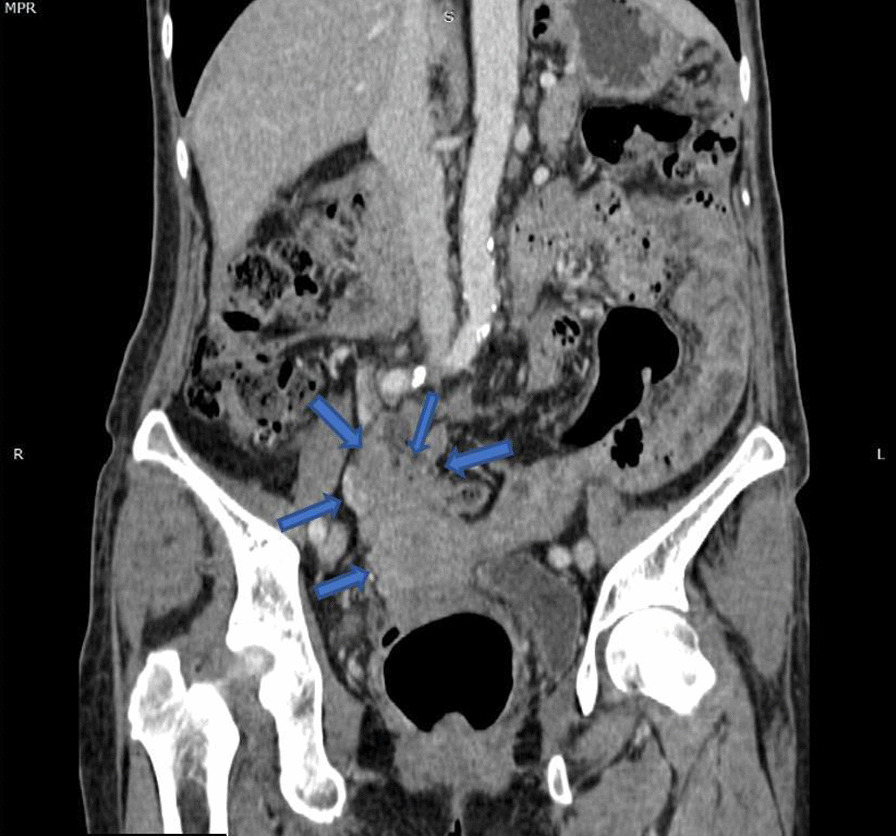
Coronal computed tomography scan image demonstrating uterine mass infiltrating the adjacent bowels and omentum (blue arrows)
